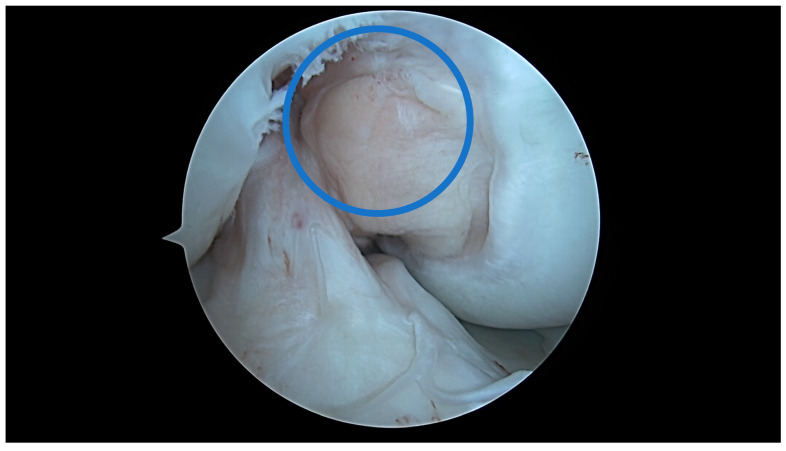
ACL–ICN relationship at 90 degrees flexion of a U-shaped notch type; blue circle = aICN area measured on the MRI.
